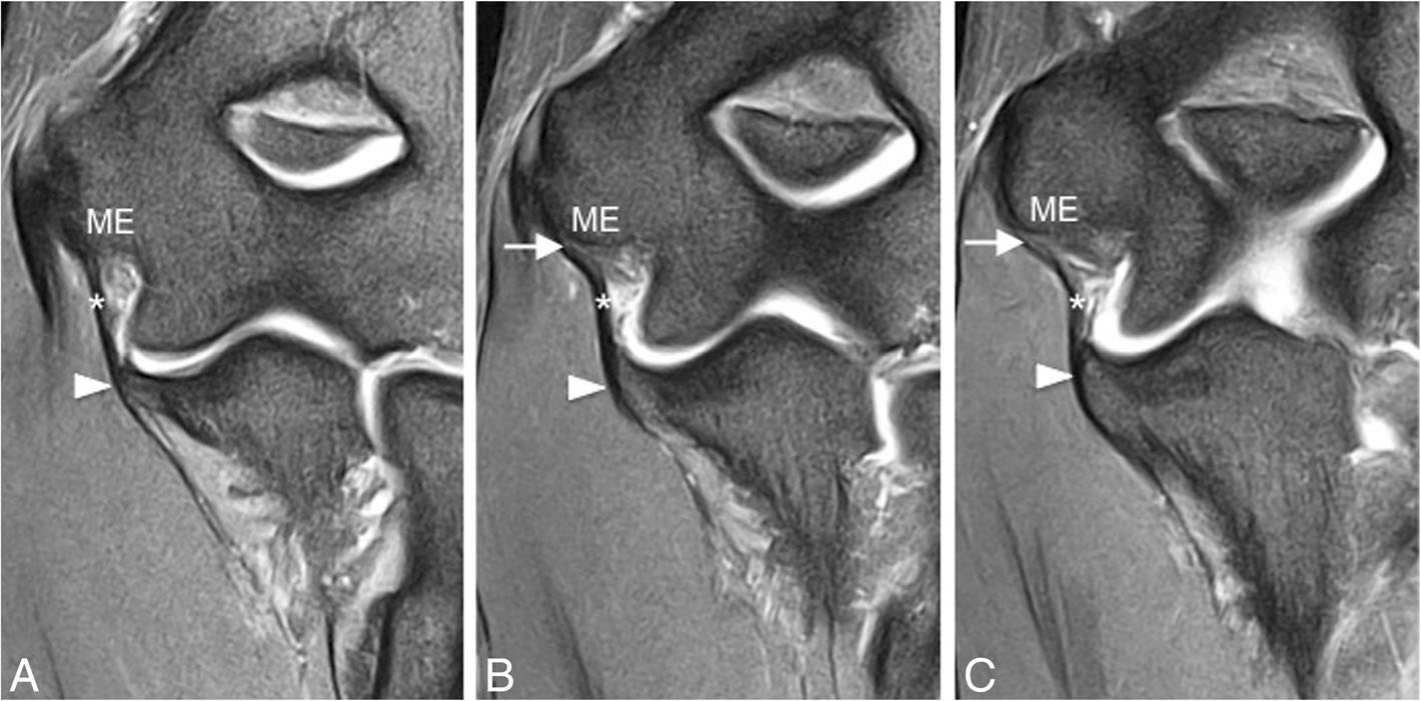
Consecutive coronal FS PD-weighted MRI (a–c) showing the intact anterior bundle of the medial collateral ligament (A-MCL) complex (white asterisks). It extends from the inferior aspect of the medial epicondyle (ME) to the sublime tubercle of the ulna (white arrowheads). Note the typical striated appearance of the normal proximal A-MCL (white arrows)
